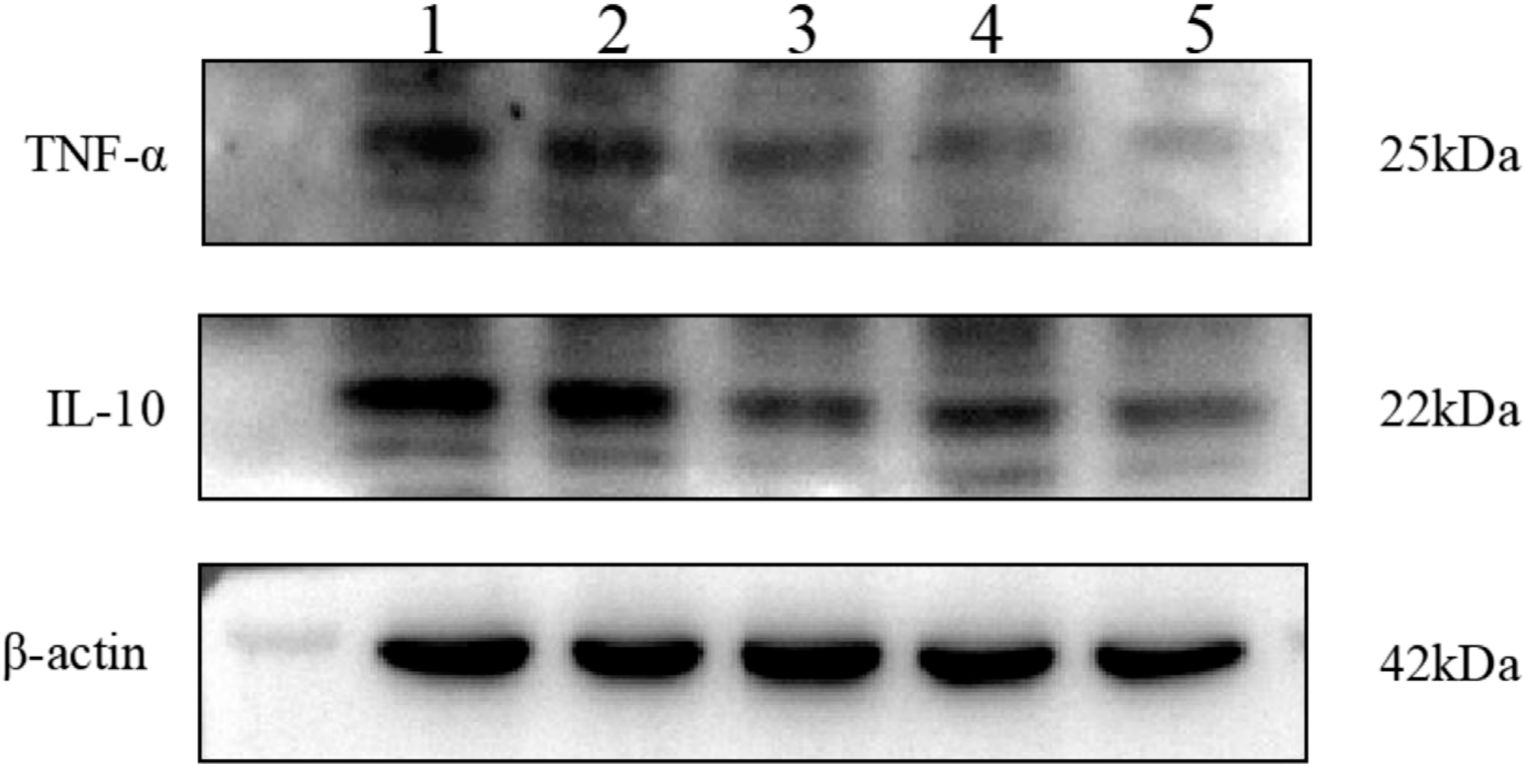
The effect of different treatment on IL-10 and TNF-α expression of HepG2 cell. 1) ultrasound; 2) FFA + ultrasound; 3) NBs + FFA + ultrasound; 4) NBs@Cu_5.4_O NPs@Res + FFA + ultrasound; 5)Apt-NBs@Cu_5.4_ONPs@Res + FFA + ultrasound.
